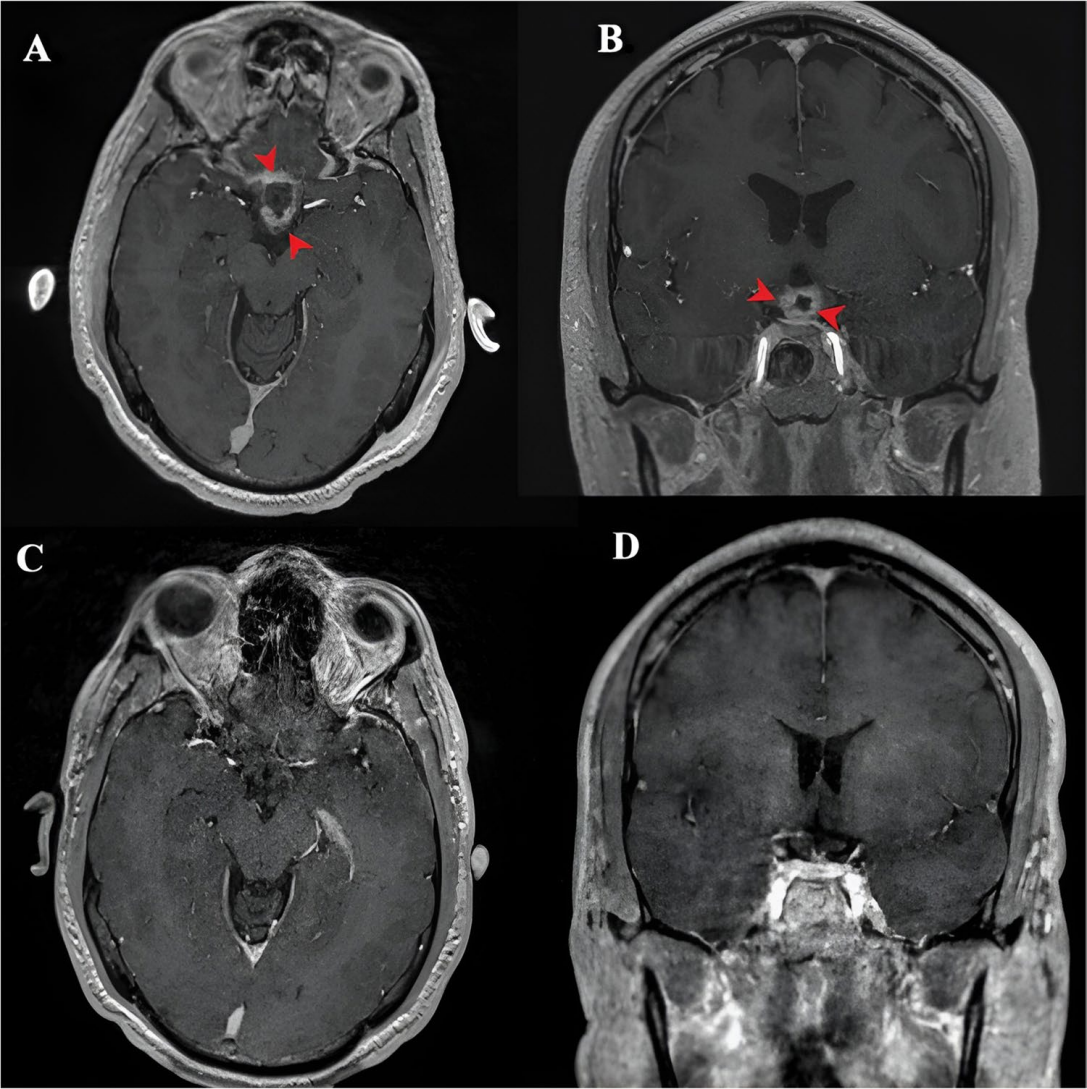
A. and B.- Immediate postoperative axial and coronal T1 with contrast MRI images of Case 1 showing residue (red arrowhead), C. and D.- Axial and coronal T1 contrast MRI images after completion of 19 months of dabrafenib + trametinib therapy showing complete resolution
